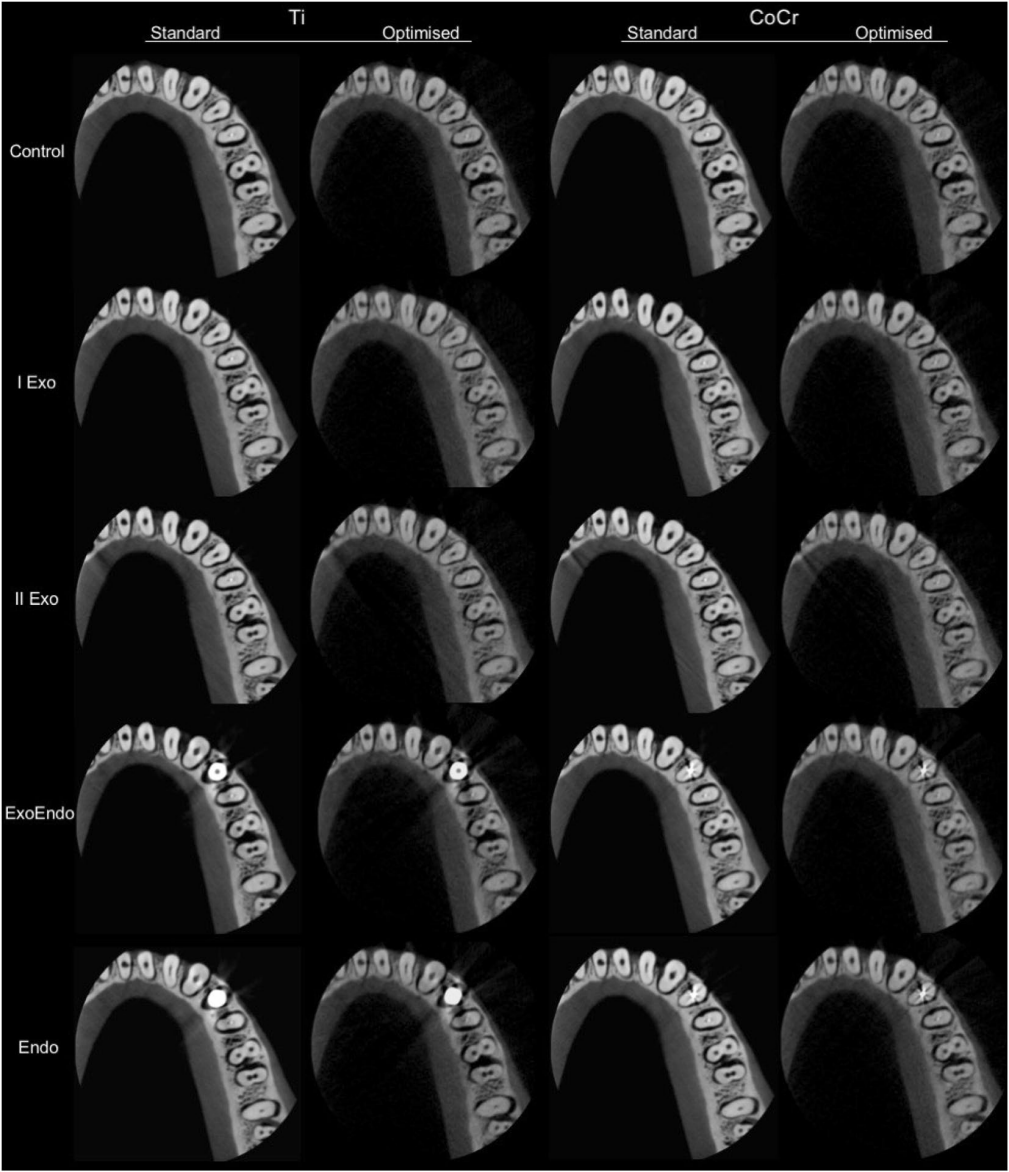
Representative CBCT axial reconstructions of the standard and optimised protocols in different dispositions of titanium implants and cobalt-chromium intracanal posts in the mandible.
